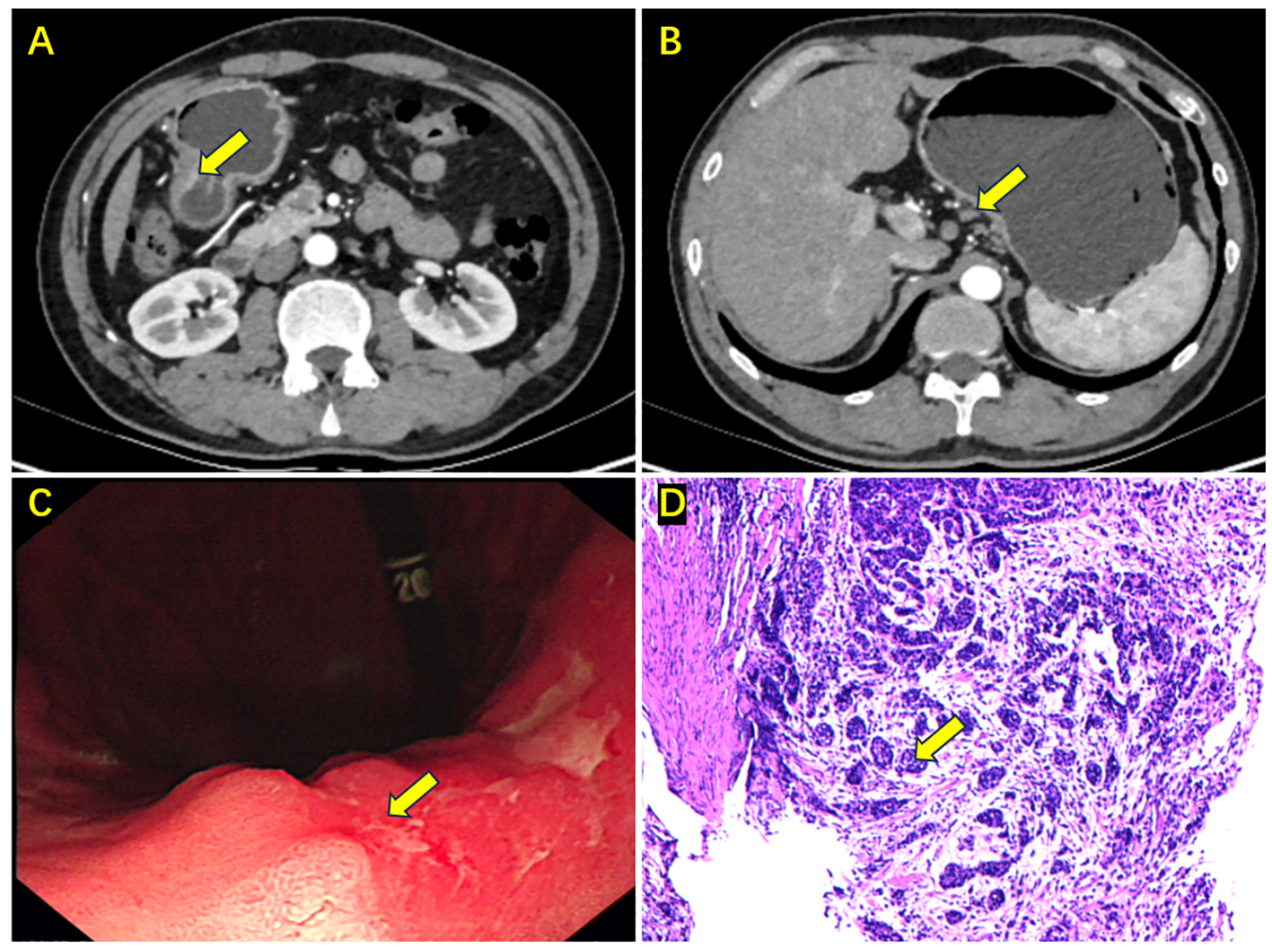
Enhanced abdominal CT, gastroscopy, and biopsy results upon initial admission. (A) Cancer in the gastric antrum (the yellow arrow); (B) Enlarged lymph node on the lesser curvature side (the yellow arrow); (C) Gastroscopy showing a mass in the gastric antrum (the yellow arrow); (D) Biopsy pathology indicating moderately differentiated adenocarcinoma (the yellow arrow).
